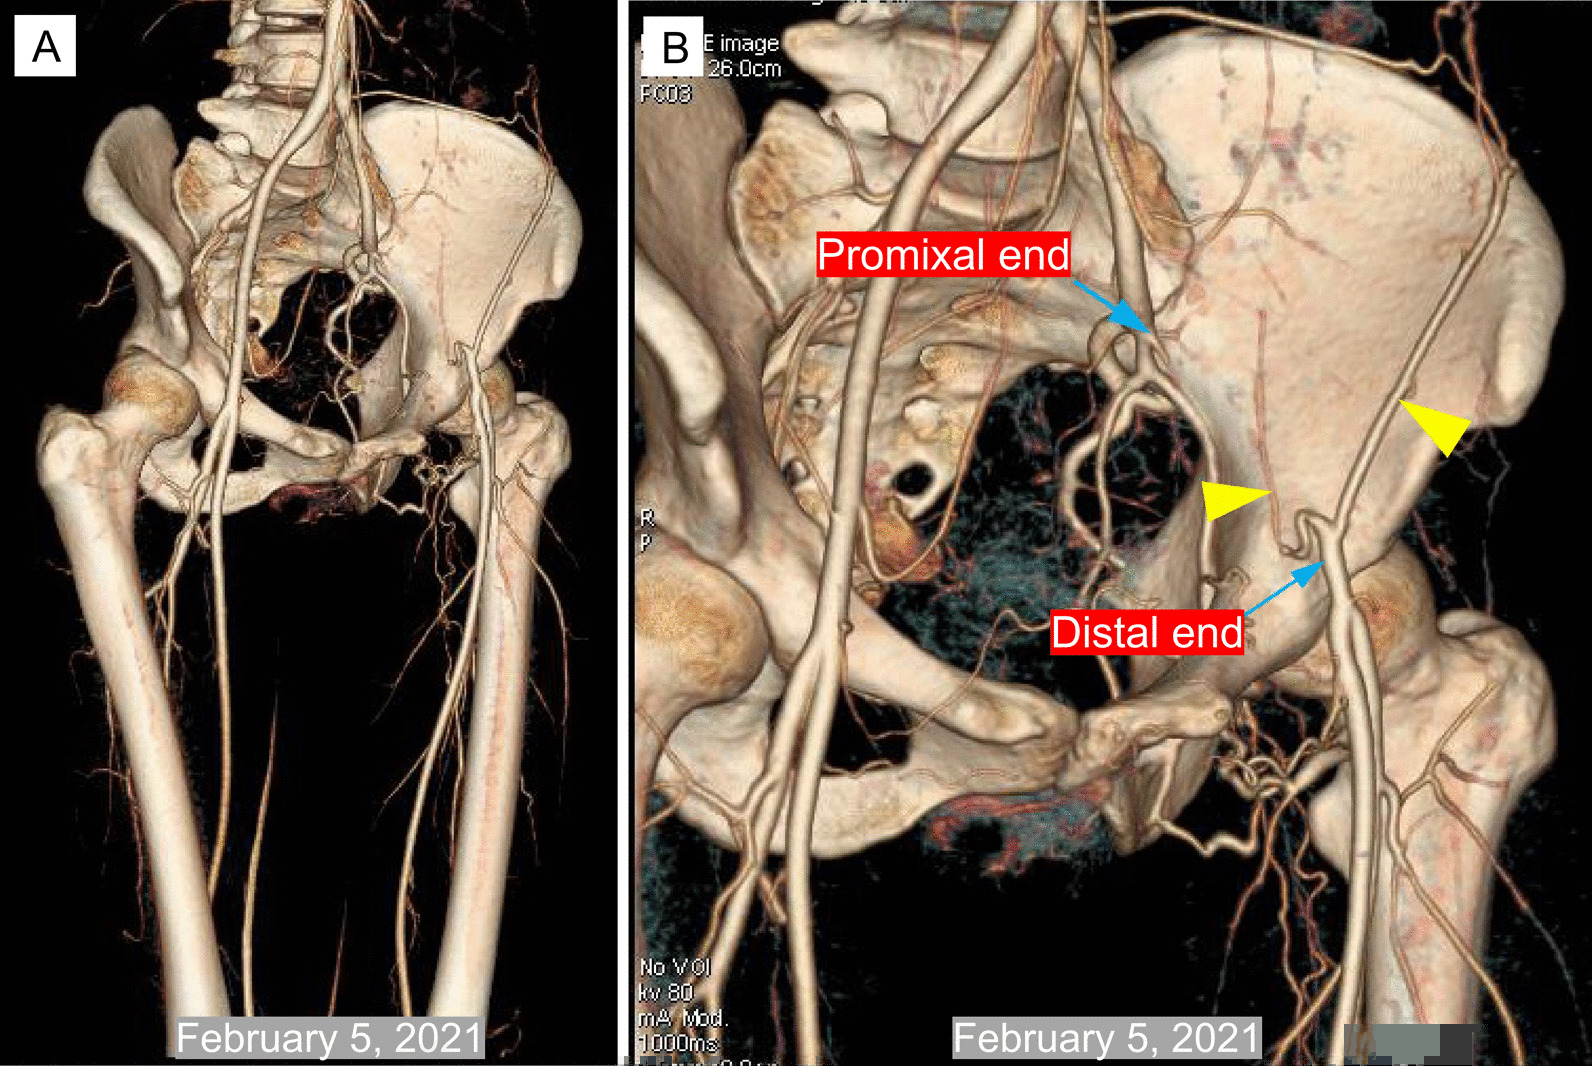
Computed tomography angiography imaging of the bilateral lower limbs on February 5, 2021 (A, CTA image of lower limb vessels; B, abnormal left external iliac artery and collateral circulation in the left external iliac artery)
